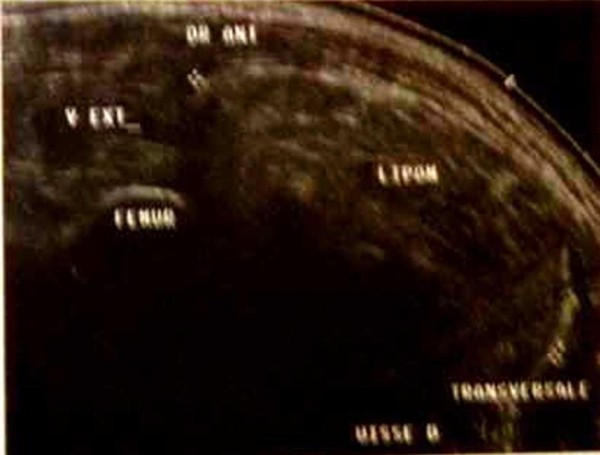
ultrasound aspect of a deep lipoma of the thigh.
